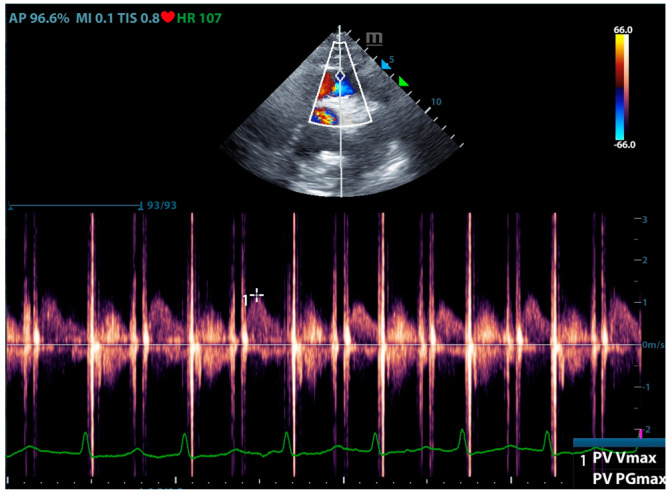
The ultrasound measurement of the maximum velocity of the pulmonary artery valve regurgitation.
